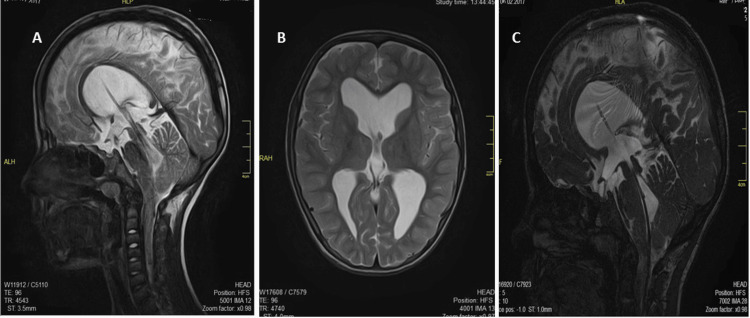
Post-surgical control at five years of age. A - MRI sagittal view showing the catheter from the lateral ventricle through the foramen of Monro, third ventricle, Silvius aqueduct, and fourth ventricle to the spinal subarachnoid space; B - Axial view showing the catheter passing through the Monro to the third ventricle; C - Cisternography, shows the drainage installed from the lateral ventricles to the posterior subarachnoid space at the level of the C5 vertebra.
